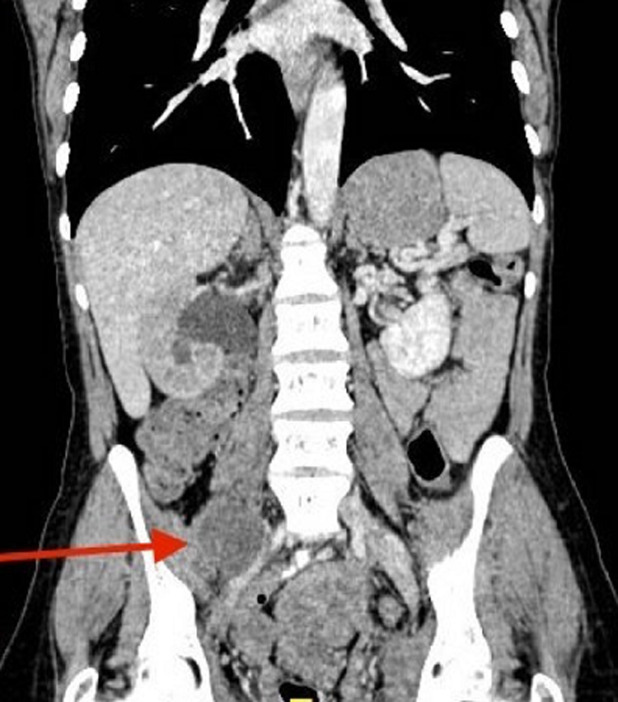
computed tomography (CT) image showing psoas abscess-like lesion with homolateral hydronephrosis
